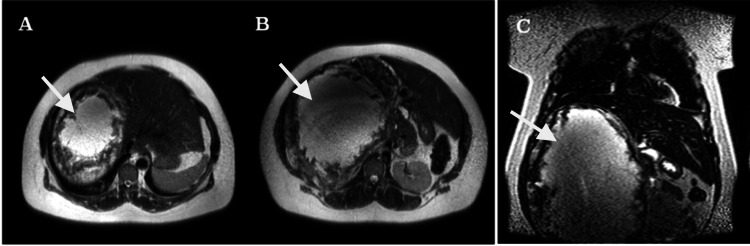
Magnetic resonance imaging axial (A-B) and coronal images (C). The image confirms the presence of a large hyperintense mass (arrows) located in the right hypochondrium.
